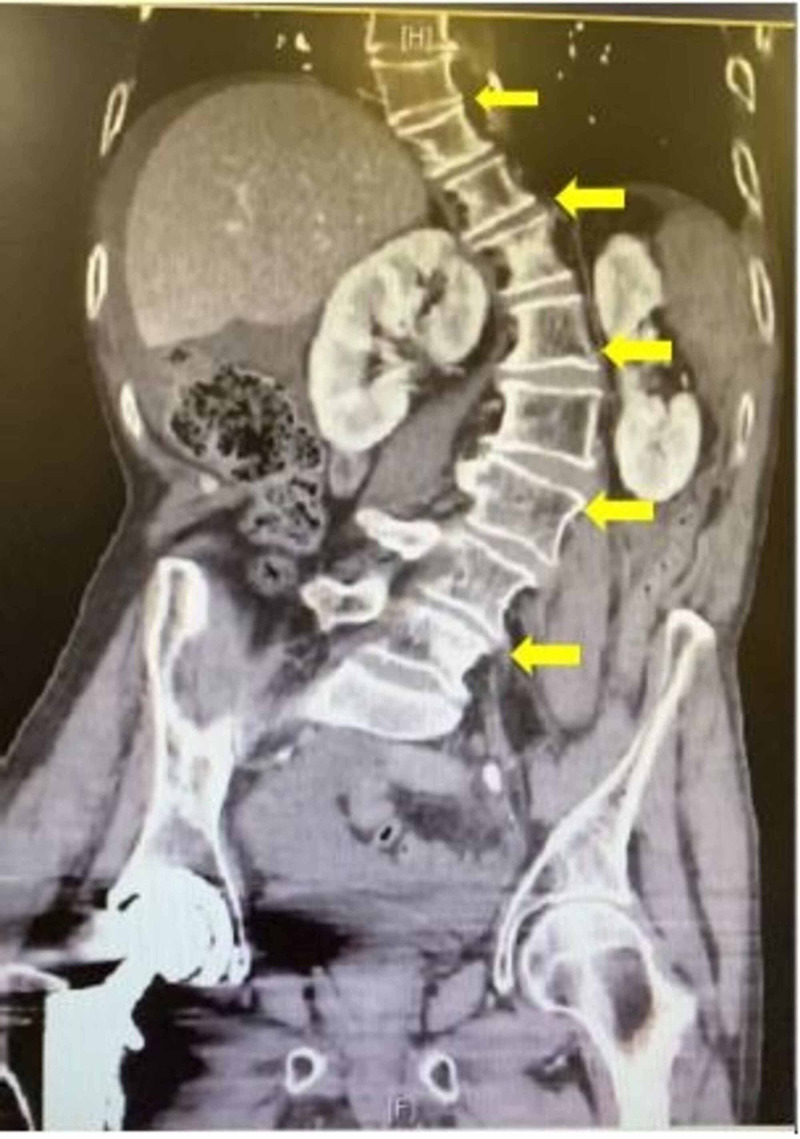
CT image depicting scoliotic vertebrae. CT, computed tomography
